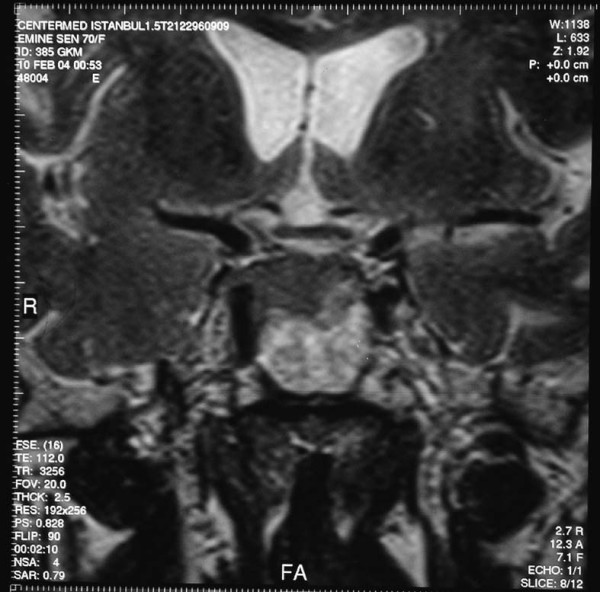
Coronal pituitary magnetic resonance imaging T2W1 with contrast agent applied.
